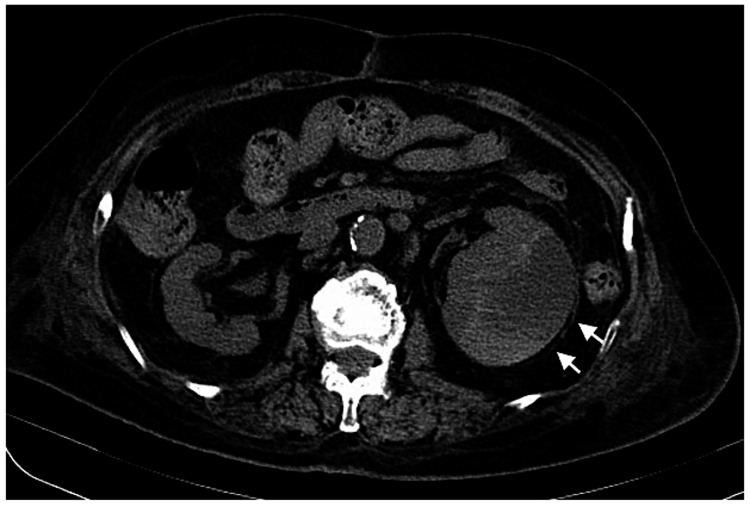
CT scan showed an enlarged left renal subcapsular hematoma (white arrows).
